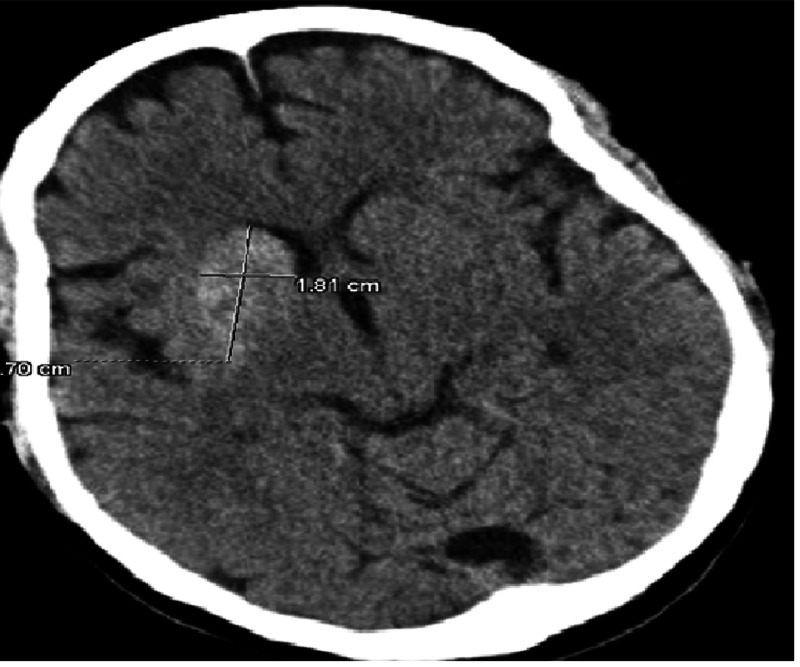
CT head showing hyperdensity (probable hemorrhage) in the right caudate nucleus head and anterior putamen CT: computed tomography
